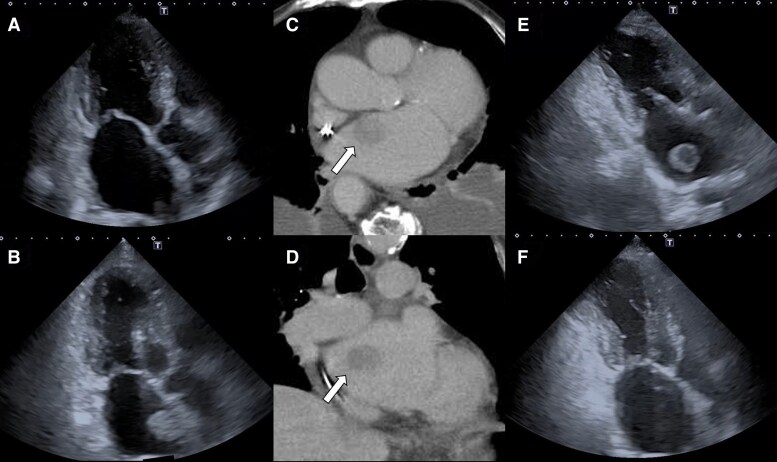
Serial transthoracic echocardiography and computed tomography findings. (*A*) No left atrial mass was observed 26 days before admission. (*B*) A mobile mass measuring 25.0 × 23.9 mm was detected in the left atrium on day 1. (*C* and *D*) Contrast-enhanced computed tomography demonstrated an intracardiac mass (white arrow). (*E*) On day 5, the mass was free-floating in the left atrium and showed morphological changes compared with day 1. (*F*) The mass disappeared from the left atrium by day 6.
